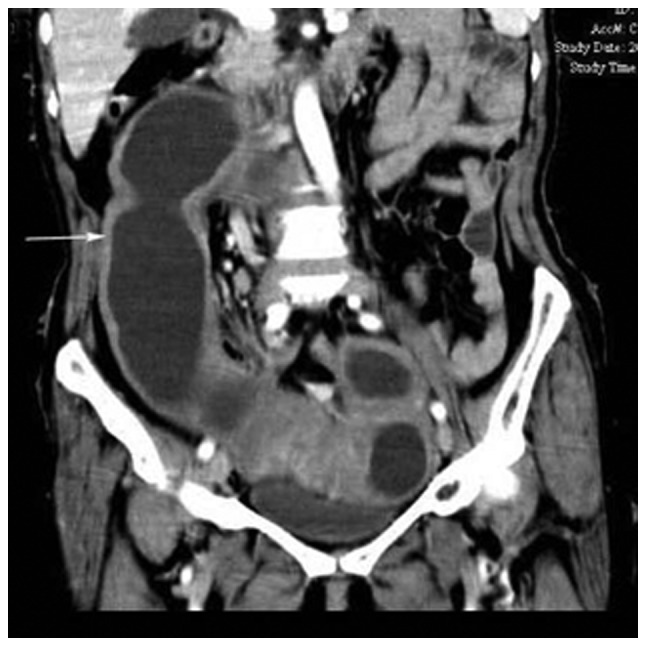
Abdominal computed tomography scan revealing a giant thick-walled tubular mass (arrow), mainly located in the right abdominal area.
